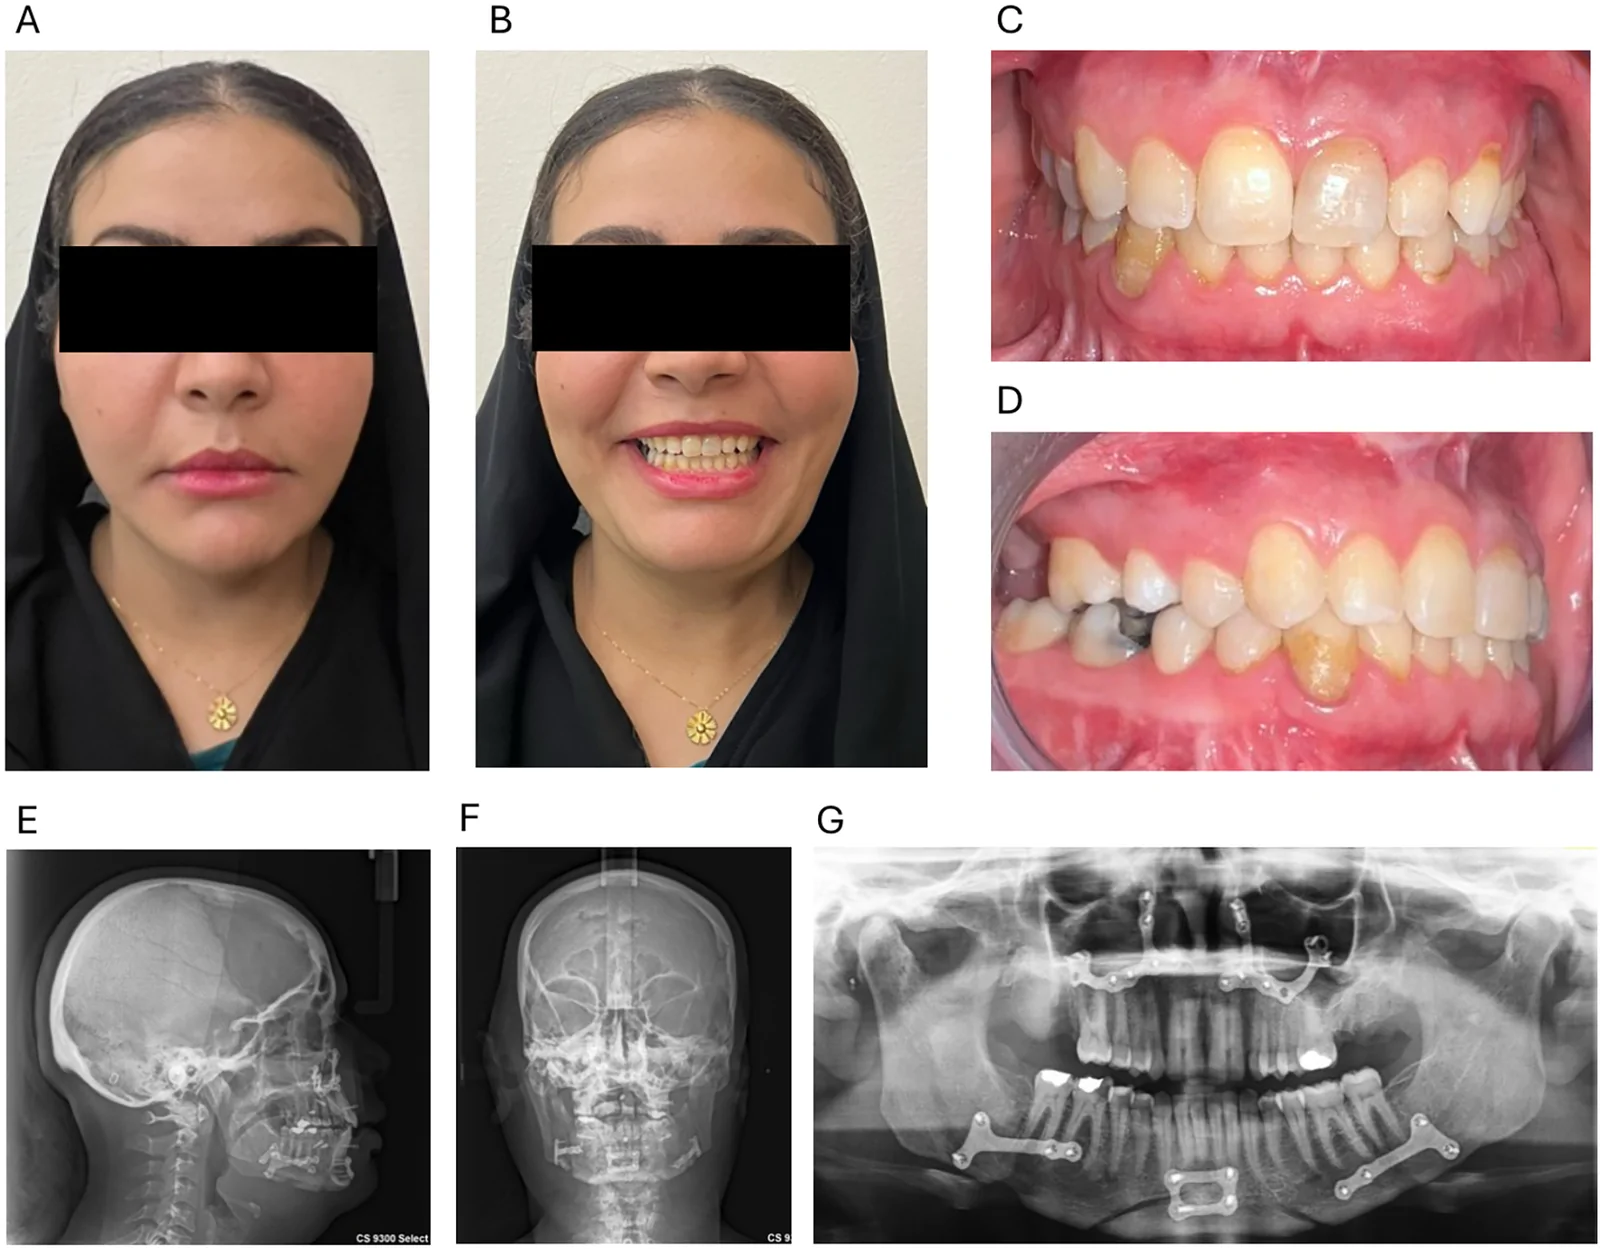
Postoperative extraoral and intraoral photos at the 6-month follow-up visit: (A) at rest, (B) smile, (C) dental midline restored with correction of occlusal canting, (D) resolution of the right posterior open bite with ideal intercuspation achieved, and postoperative radiographs obtained on postoperative day 4: (E) lateral cephalometric, and (F) posteroanterior view. (G) Orthopantogram acquired three months postoperatively.
